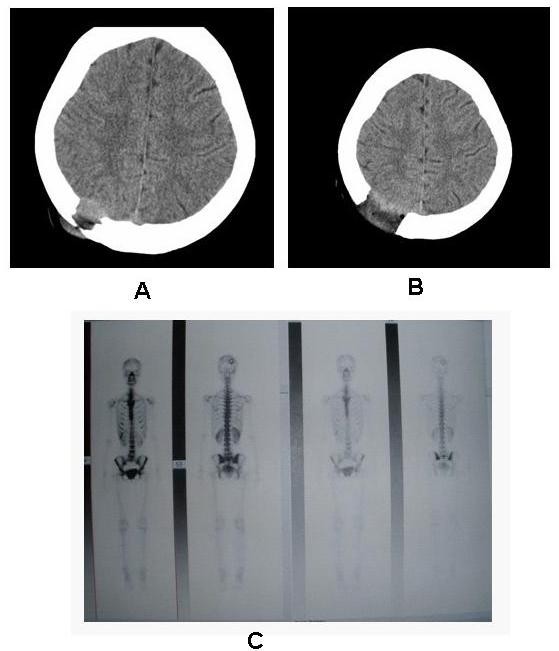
Metastatic tumor of the right parietal bone. A: CT scan of the brain shows that the right parietal bone is ruined before the craniectomy. B: CT scan of the brain after craniectomy. C: The emission-computed tomography scan shows an abnormal distribution of radioactivity in the right parietal bone area; the sparse distribution of radioactivity was central; peripheral distribution of radioactivity uptake before craniectomy.
